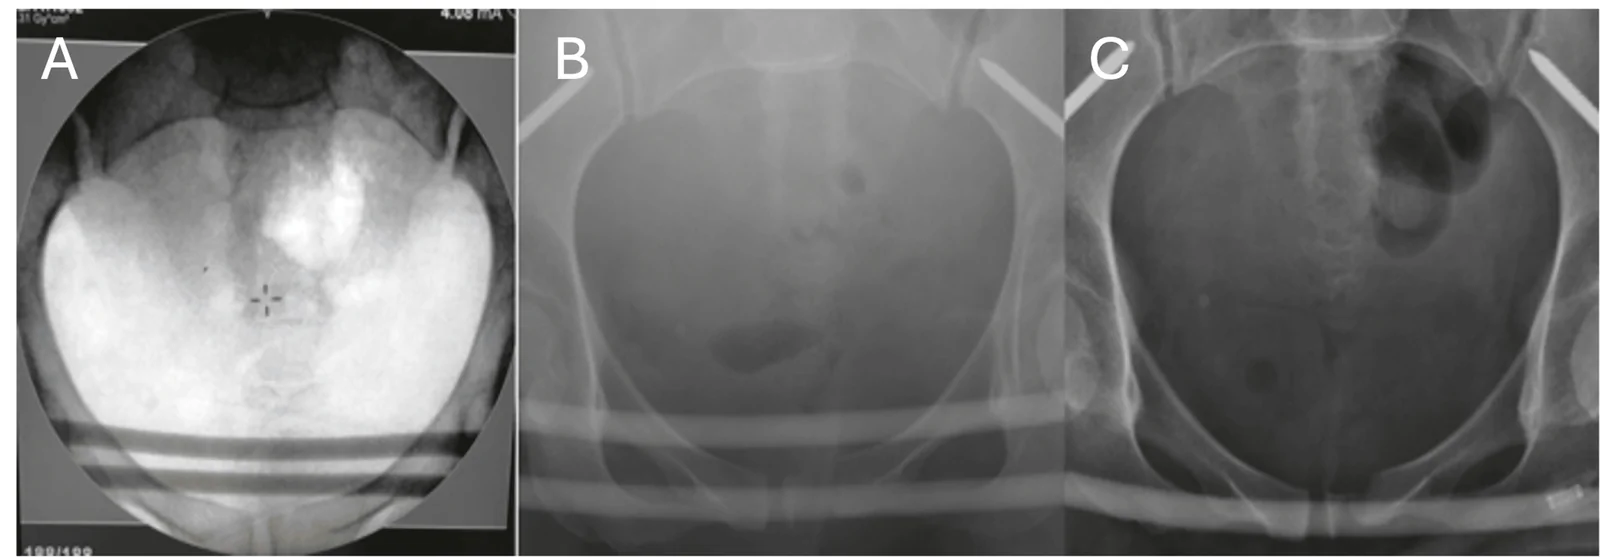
Sequential pelvic inlet radiographs showing reduction over time (A) Intraoperative reduction. (B) Immediate postoperative radiograph. (C) Two-week follow-up, showing maintained pelvic reduction with the external fixator in situ.
